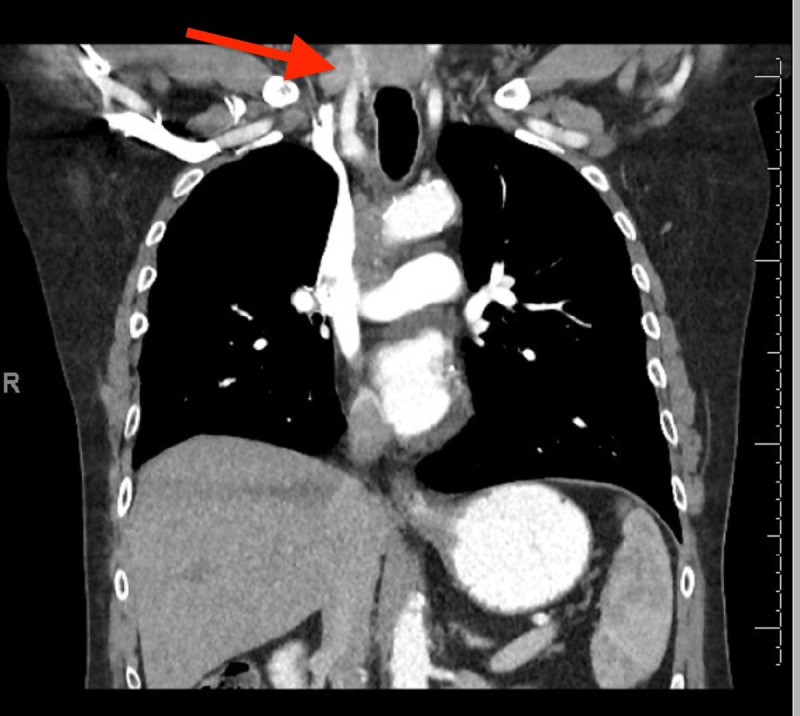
CT scan of the chest with contrast in the mediastinal window and coronal plane showing an enlarged right supraclavicular lymph node.
